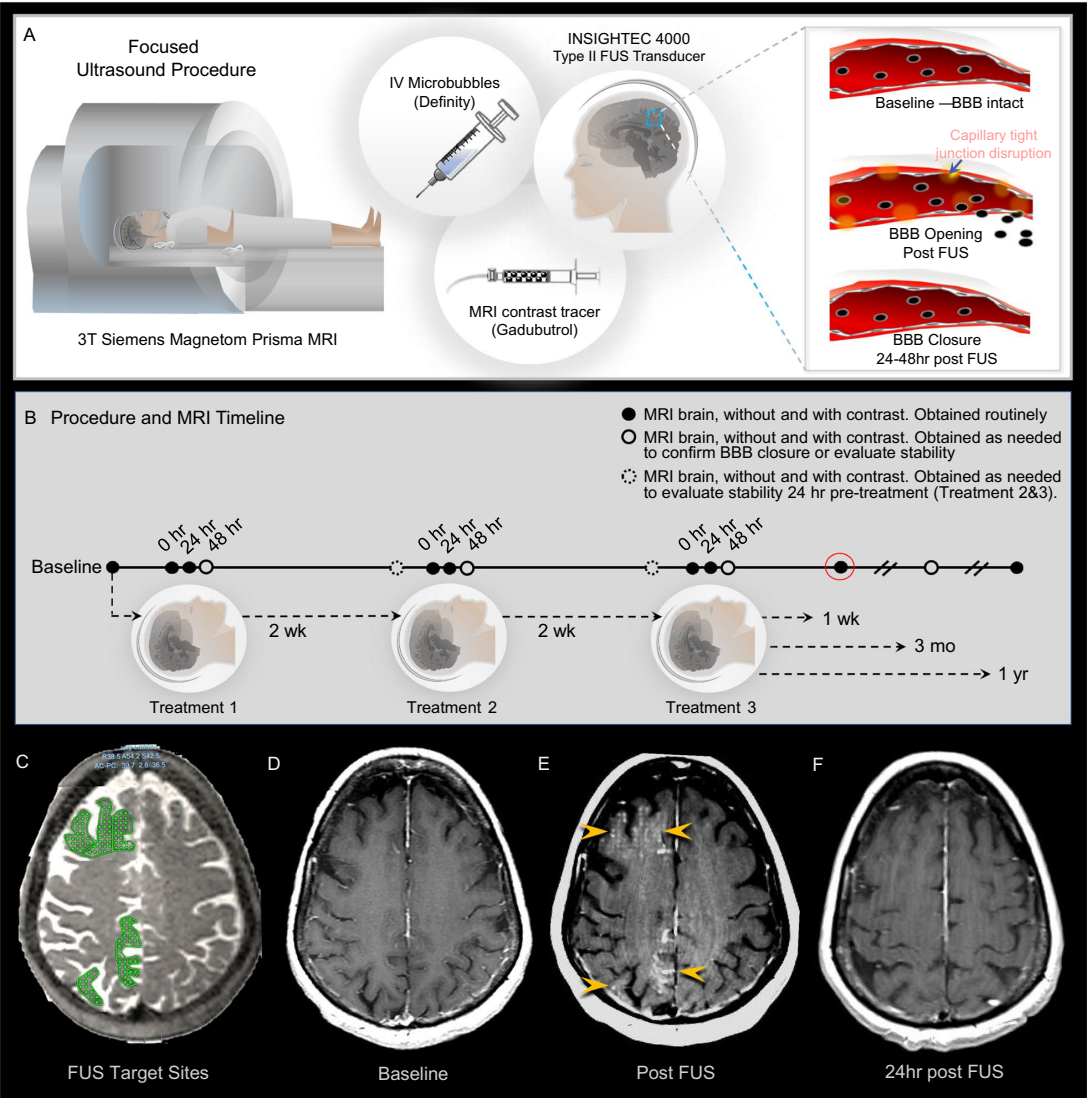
FUS procedure for targeted transient blood–brain barrier opening. The study was conducted using the Insightec Type II FUS transducer in combination with Definity® microbubbles A. Imaging was acquired using a 3-Tesla Siemens MRI scanner and gadobutrol contrast tracer in 8 volunteer subjects with early Alzheimer’s disease. The treatment and MRI timeline is shown B. FUS target sites within the frontal and parietal lobes are shown in green C. MRI scans show blood–brain barrier (BBB) opening and closure within these targeted brain volumes in a 73-year-old woman with Alzheimer’s disease D–F. Post-contrast T1 weighted images, at baseline D, immediately after FUS treatment E, and 24 h after treatment F show contrast tracer extravasation into parenchyma (arrowheads, E) due to focal BBB opening at the prescribed treatment sites. The BBB is closed at 24 h post FUS intervention F, with no contrast enhancement seen at target sites following repeat gadobutrol contrast tracer administration. *BBB* blood–brain barrier, *Hr* hour, *IV* intravenous, *Mo* month, *Tx* treatment, *Wk* week, *Yr* year
